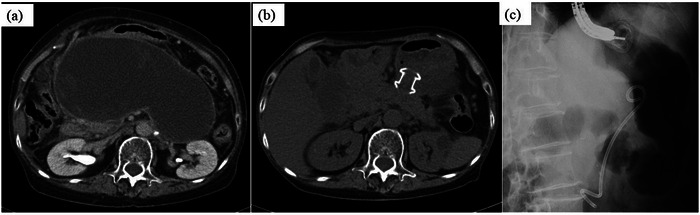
Contrast‐enhanced computed tomography. A huge walled‐off necrosis is in the abdominal cavity. (a) Abdominal plain computer tomography after lumen‐apposing metal stent implantation and treatment showing reduction of walled‐off necrosis. (b) The lumen‐apposing metal stent was removed. A double pigtail plastic stent (7Fr, 7 cm) was implanted.
